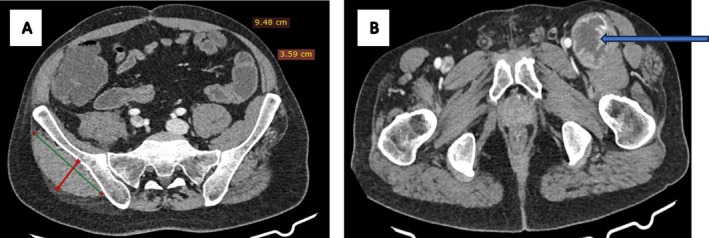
A and B are contrasted CT scans of the pelvis, axial slices showing ovoid and round heterogeneously enhancing soft tissue density masses in the right gluteal (A) and left inguinal area (blue arrow in B).
